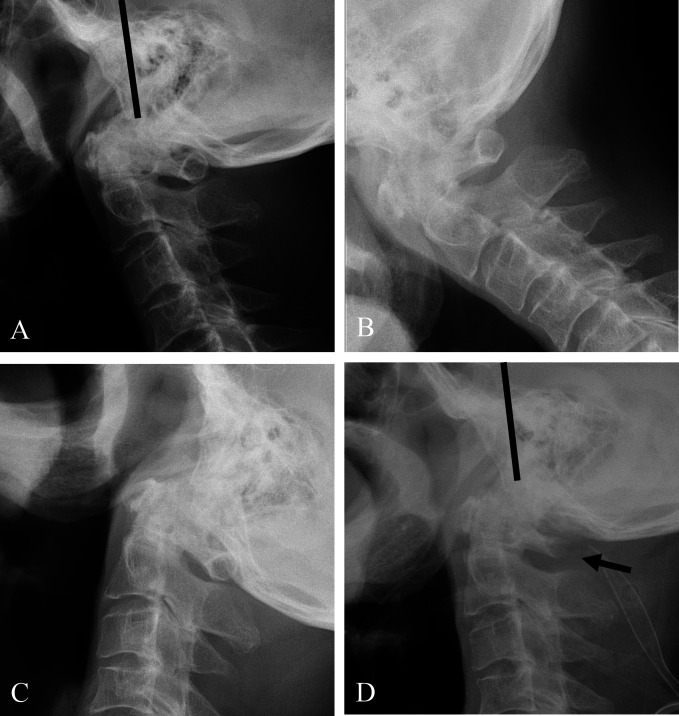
Preoperative lateral radiograph (A–C) of the cervical spine showing running of Wackenheim line (dark line) behind the dens. Postoperative lateral radiograph (D) of the cervical spine demonstrating laminectomy of the C1 posterior arch (dark arrow).
